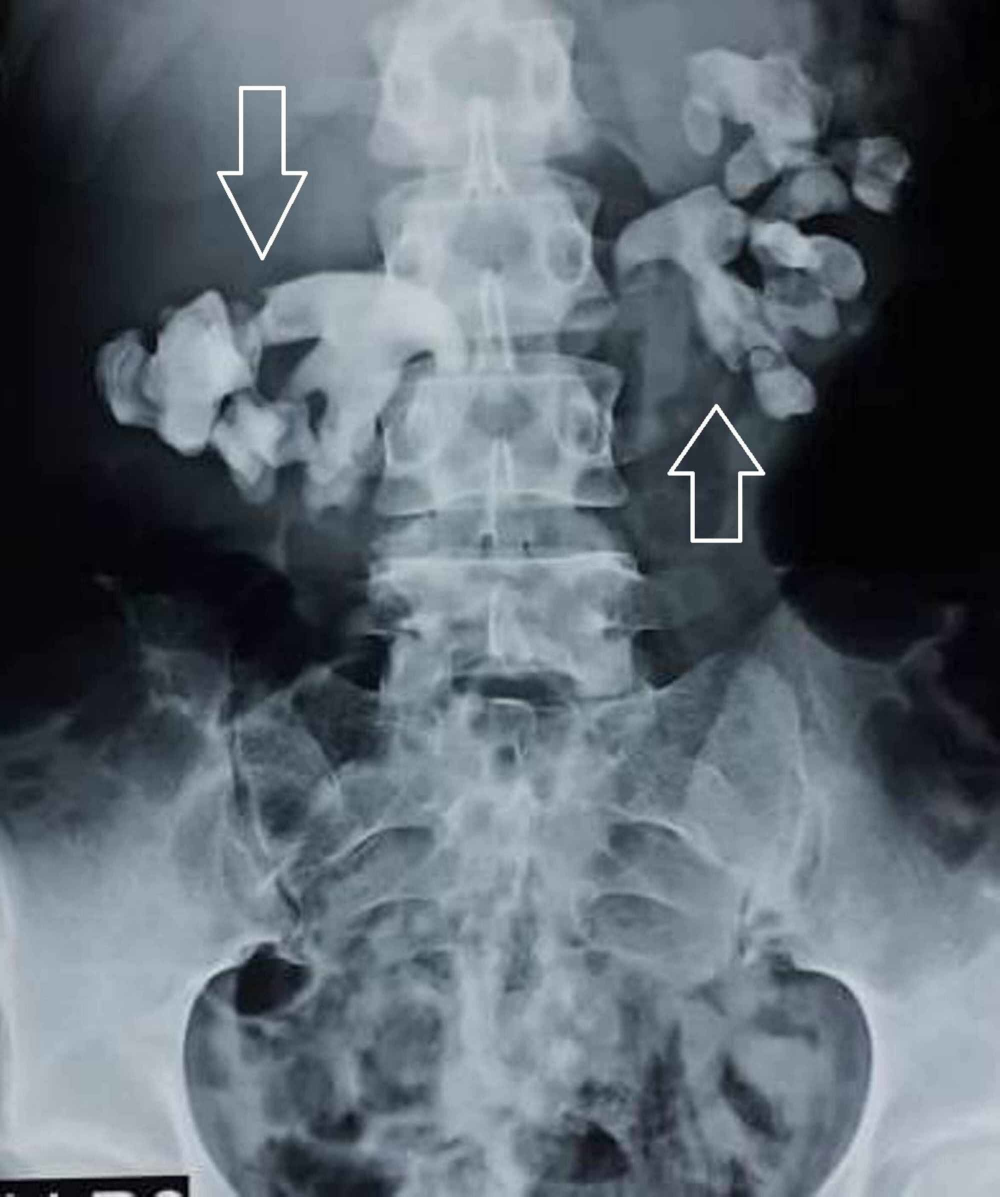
X-ray kidney ureter bladder showing bilateral staghorn renal calculi (white arrows).
